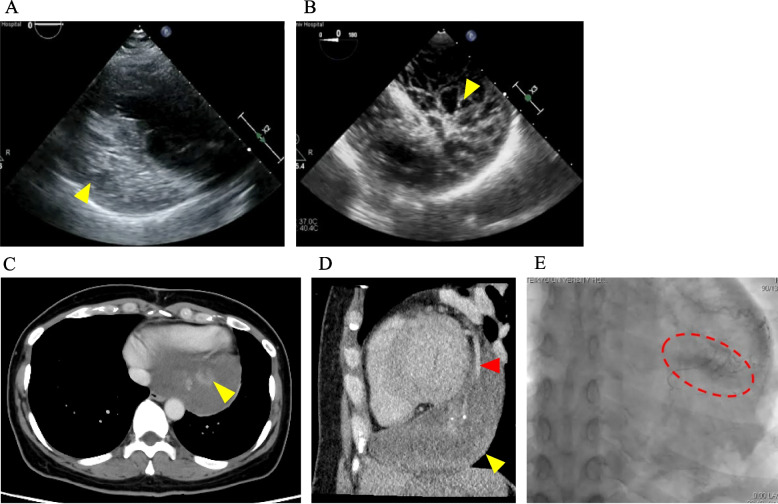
Preoperative findings. A Transthoracic echocardiography. B Transesophageal echocardiography. C, D Computed tomography. A tumor is shown as a yellow triangle and arterial inflow is shown as a red triangle. E Coronary angiography. Pooling of contrast agent is shown as a red circle
